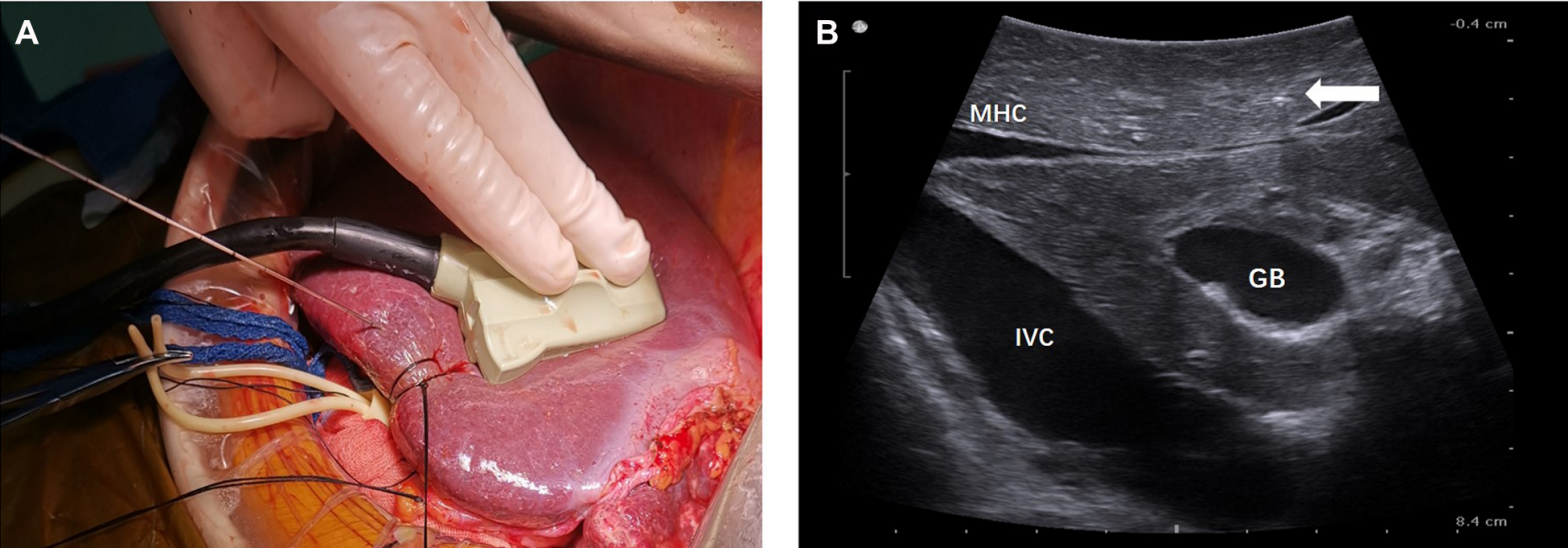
(A) Intraoperative view showing insertion of a 21-G needle into the liver toward MHV under ultrasonography guidance after ligation of the left portal vein and liver artery. (B) Intraoperative ultrasonography image showing the resection plane determined according to the MHV and the inserted needle (white arrow). The IVC may also be included in the resection plane. IVC, inferior vena cava; MHV, middle hepatic vein; GB, gallbladder.
